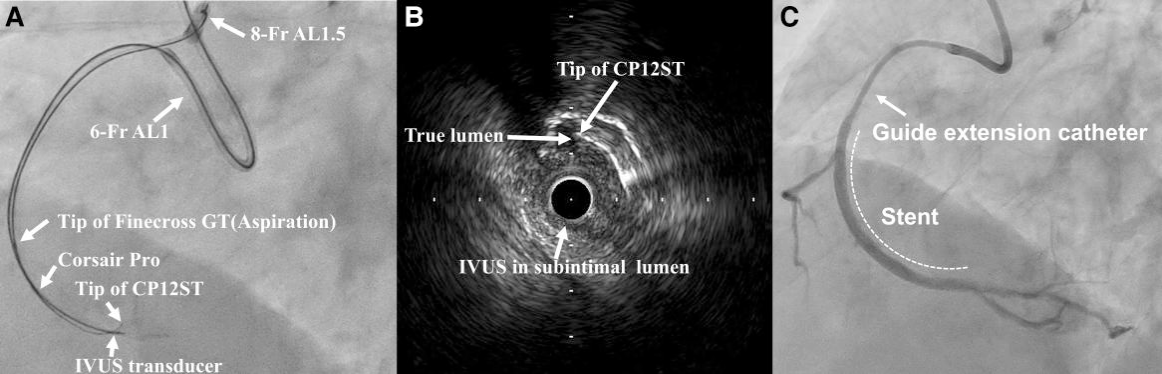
Successful reentry using intravascular ultrasound–guided tip detection under the subintimal transcatheter withdrawal technique. (*A*) Intravascular ultrasound–guided tip detection antegrade dissection and reentry under the subintimal transcatheter withdrawal technique. (*B*) Intravascular ultrasound image of successful penetration from the subintimal lumen to the true lumen using Conquest Pro 12 Sharpened Tip wire. (*C*) Final angiogram. CP12ST, Conquest Pro 12 Sharpened Tip; IVUS, intravascular ultrasound.
